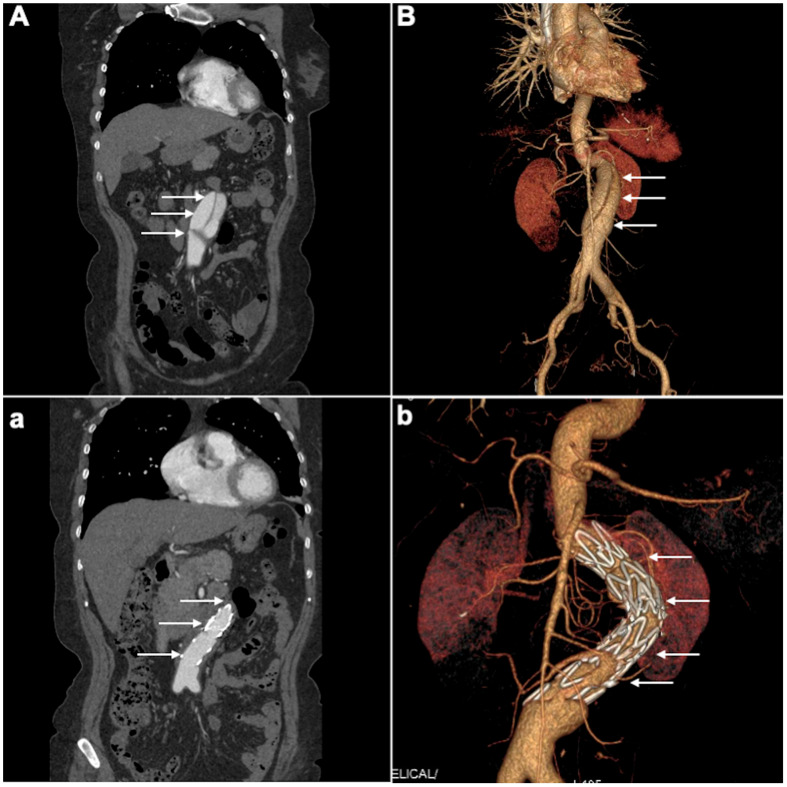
(A) pre-EVAR CTA intimal flap separates true and false lumens within the abdominal aorta. (a) post-EVAR CTA successful stent-graft coverage of entry tear with absence of false lumens opacification. (B) Pre-EVAR 3D-CTA visualizes the SIAAD extending through the infrarenal aorta with evidence of a dissecting aneurysm and a prominent false lumen. (b) Post-EVAR 3D-CTA complete exclusion of the false lumen and successful aneurysm repair, with the endovascular stent graft visible. White arrows denote the intimal flap, false lumen, and stent-graft deployment. 3D-CTA, Three-dimensional Computed Tomography Angiogram; SIAAD, spontaneous isolated abdominal aortic dissection.
